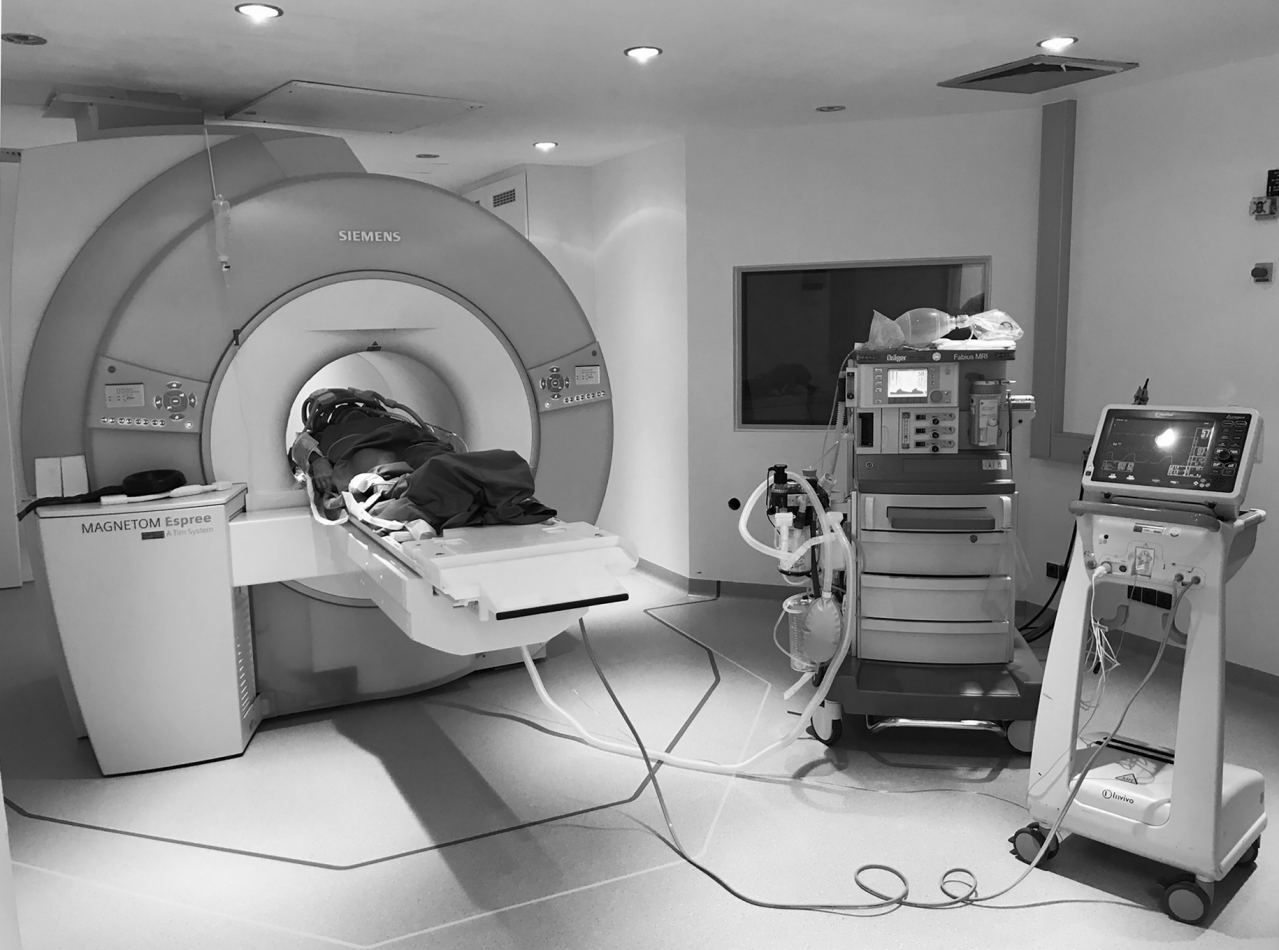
Setup of anesthesia equipment in the iMRI scanner. The MR-capable ventilator (Dräger Fabius MRI, Drägerwerke, Lübeck, Germany) is positioned outside the 30 mT area (dark line) while the monitoring hardware has to be kept outside the 20 mT area (light yellow line).
